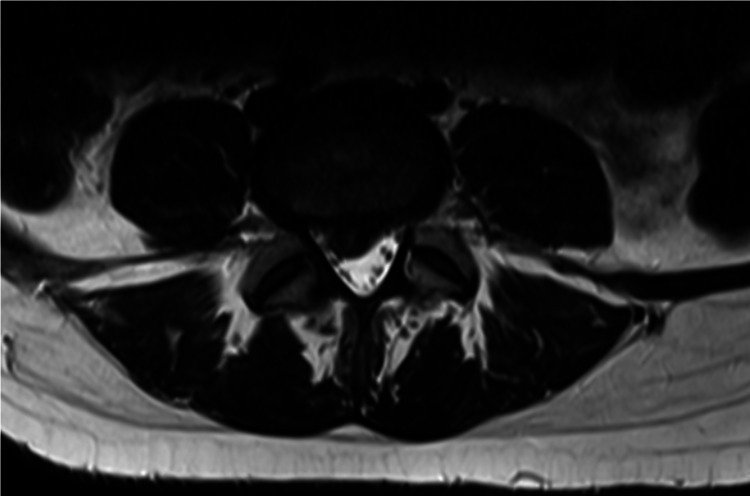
Axial MRI of the lumbar spine. Central disc extrusion extending to the right lateral recess and causing compression of the descending nerve roots.
